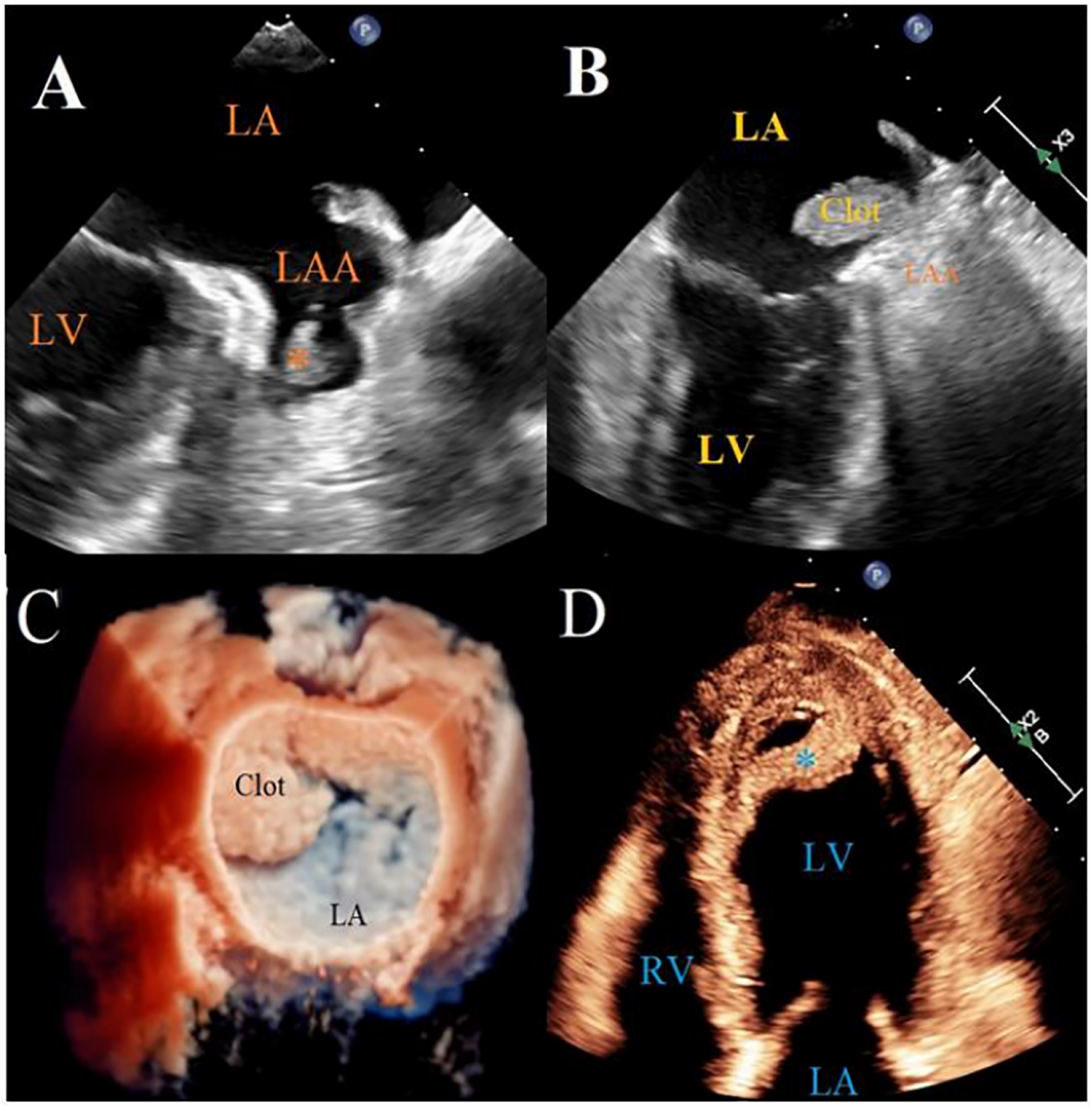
Left atrial appendage thrombus on TTE and TEE. (A) TEE image of a small LAA thrombus * in patient with AF. (B,C) 2D and 3D TEE reconstruction of a large thrombus protruding from LAA into the left atrium in a patient with factor V Leiden and AF. (D) TTE image of an apical LV thrombus *, detaching from the LV apex in a patient with acute myocardial infarction. TEE, transesophageal echocardiography; TTE, transthoracic echocardiography; LAA, left atrial appendage; LV, left ventricle; AF, atrial fibrillation.
